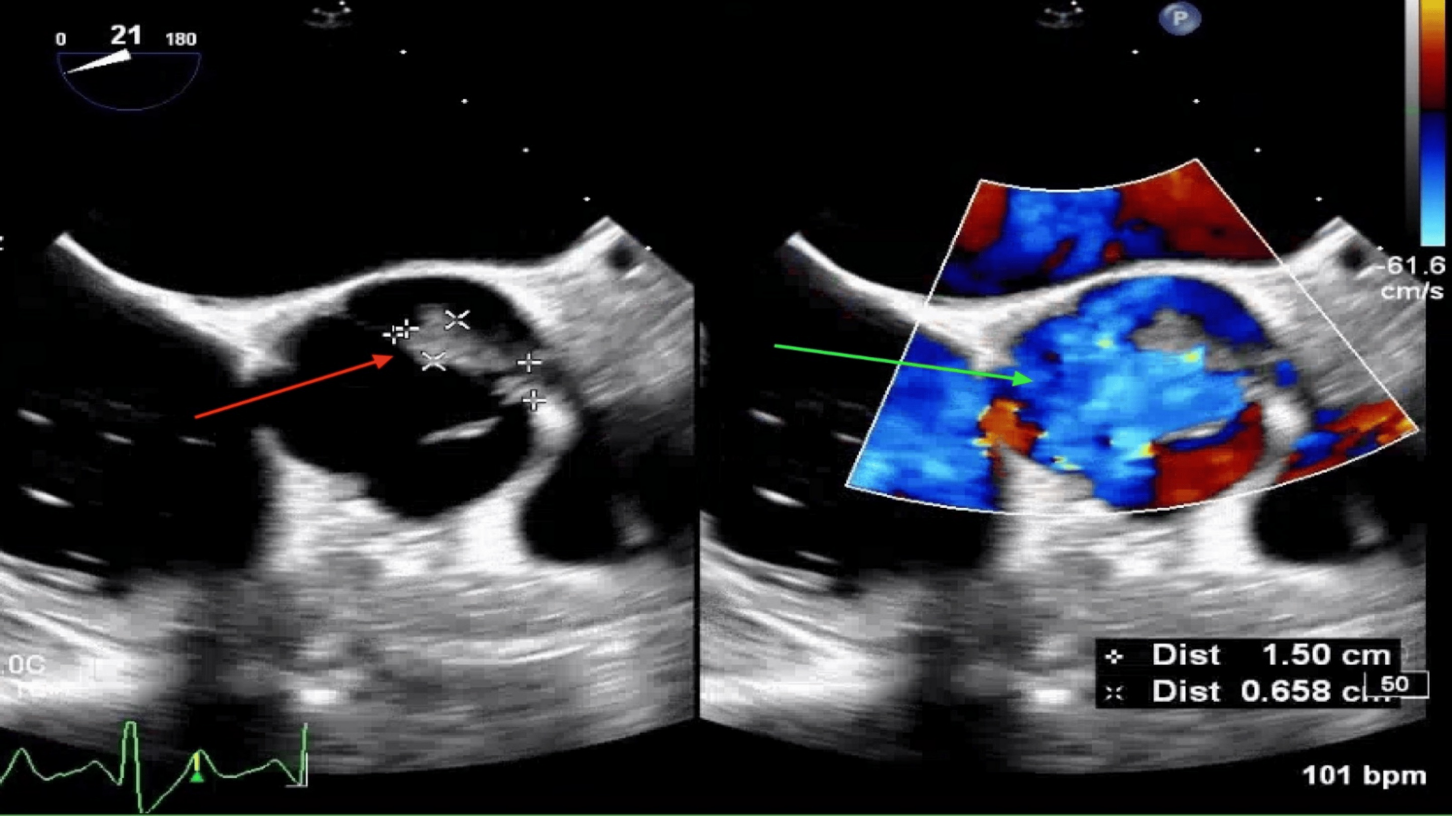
Transesophageal echocardiogram revealing a 15 × 6 mm vegetation at the tip of the left coronary cusp of the aortic valve (red arrow) and severe aortic insufficiency (green arrow).
